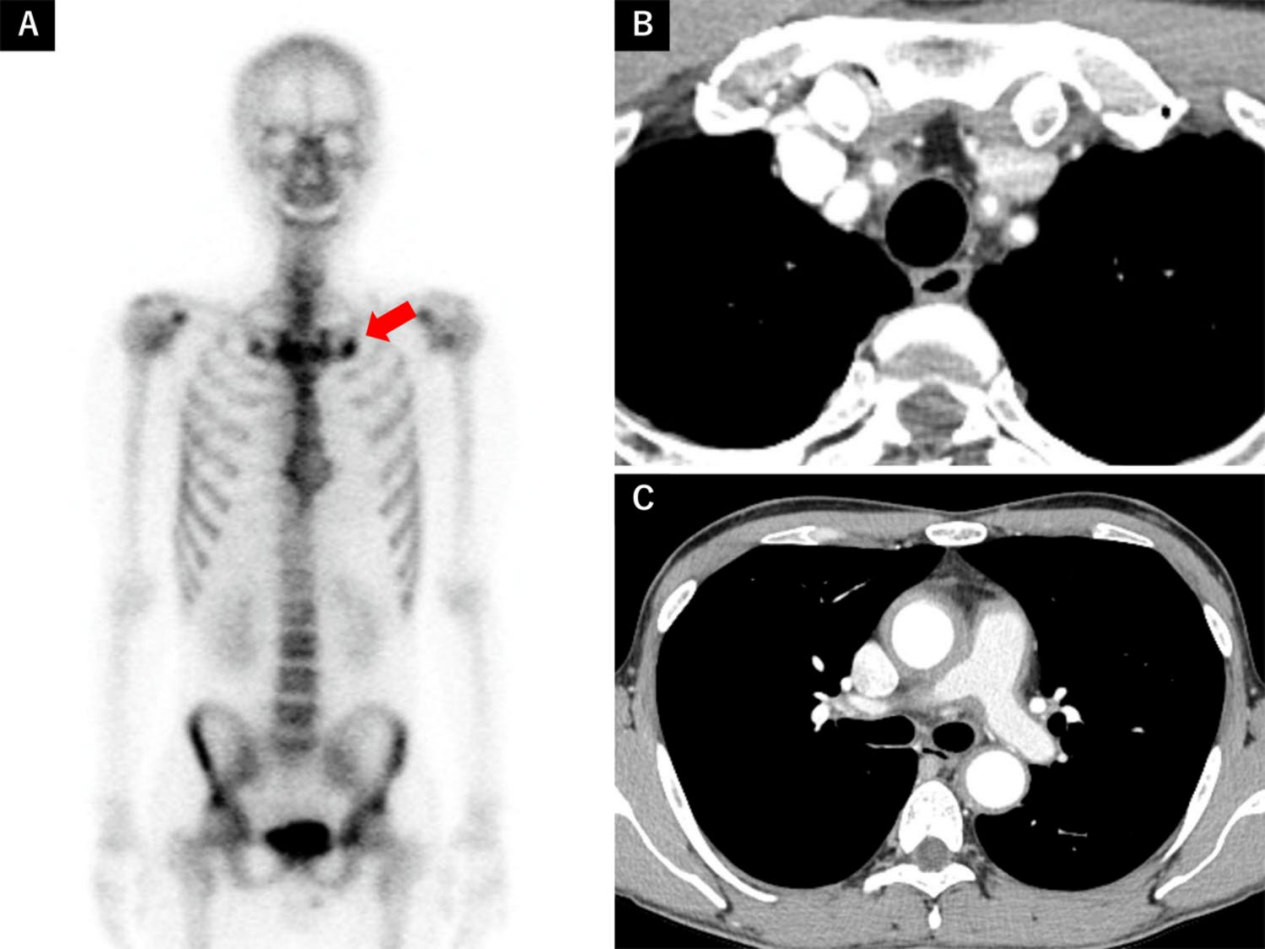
A 99 m-Tc bone scintigraphy showing tracer accumulation in the first sternal rib joint. B C Chest CT demonstrating circumferential wall thickening with a contrast effect of the ascending to descending aorta, bilateral common carotid arteries, the left subclavian artery, and the main trunk of the pulmonary artery
